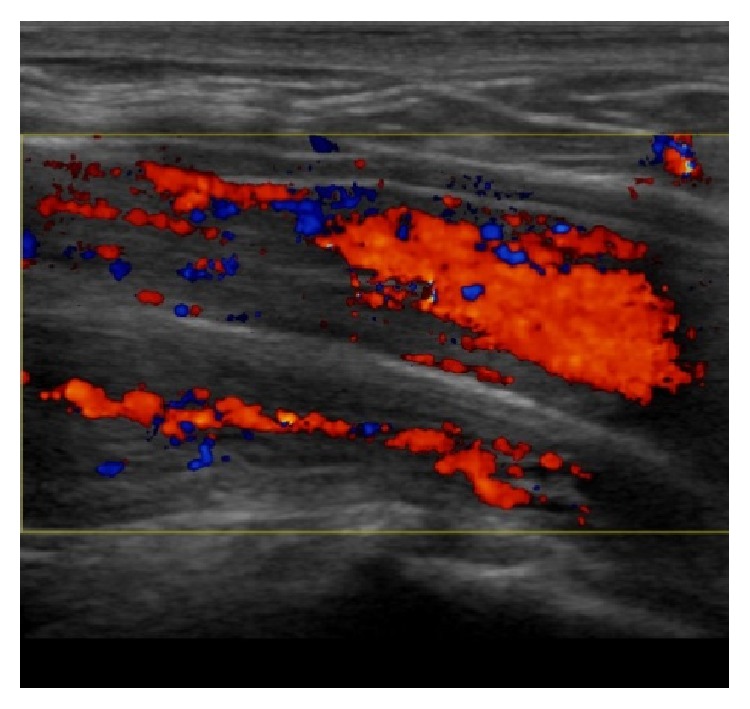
Midperineal elevation test: relaxed state with elevation. Increased Doppler activity during elevation within corpus spongiosum (CS).
